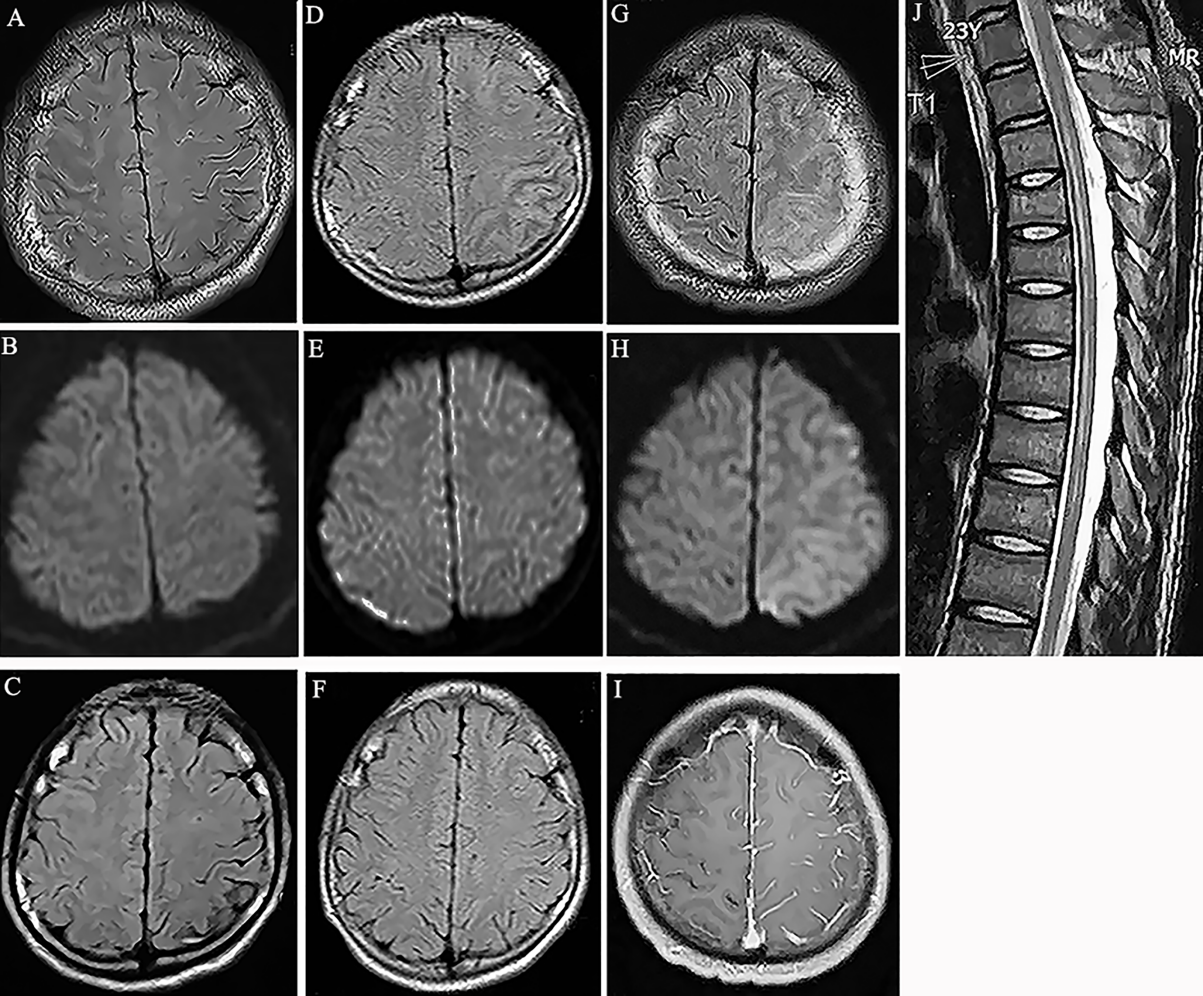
(A) Fluid attenuation inversion recovery (FLAIR) image showed hyperintensity in the cortical region of right frontal and parietal lobes without white matter involvement (Case 1). (B) Diffusion weighted imaging (DWI) showed no obvious signal change in the cortex (Case 1). (C) MRI scan showed the abnormality disappeared at the follow-up scan (Case 1). (D) Hyperintensity in the cortical regions of left parietal lobes in MRI FLAIR images 7 years before this episode (Case 1). (E) DWI showed no obvious signal change in the cortex (Case 1). (F) MRI scan showed the abnormal lesions disappeared at the follow-up scan (Case 1). (G) Hyperintensity in the cortical regions of left frontal and parietal lobes in MRI FLAIR images (Case 2). (H) DWI showed mild hyperintensity in the involved cortical regions (Case 2). (I) Gadolinium enhanced T1-weighted image showed meningeal linear enhancements in the sulci of left brain lobes (Case 2). (J) T2-weighted image showed hyperintensity in the spinal cord from thorax 2 to 9 segments. (Case 2).
